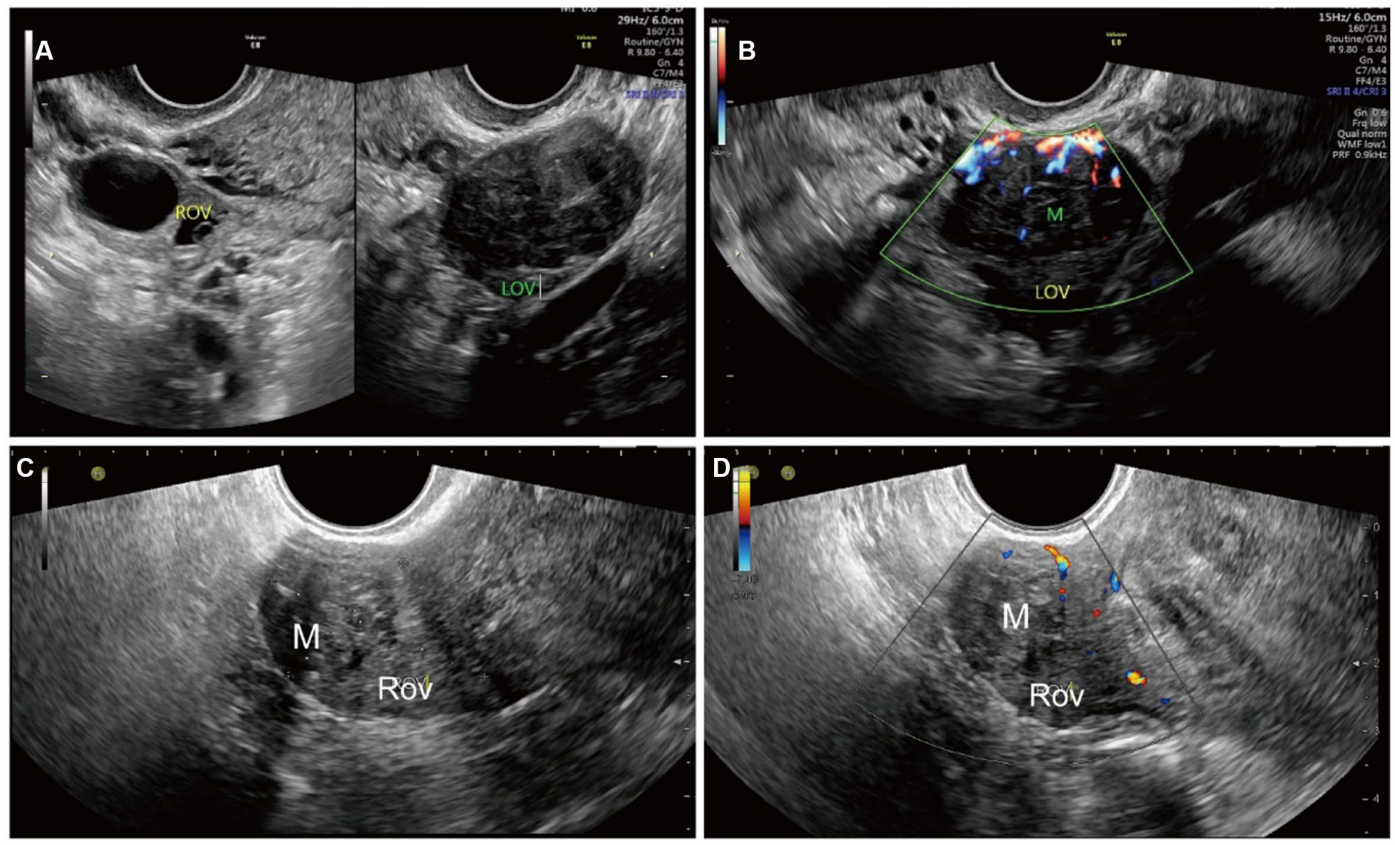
Ultrasonographic imaging of an OL localized inside the ovary in case 4 (A,B) and case 2 (C,D). (A) A well-circumscribed, heterogeneous ovarian mass was detected by transvaginal ultrasound. The mass was hypoechogenic and more hypoechoic than the surrounding ovary. (B) Strip-color flow signals in and around the mass were detected by color Doppler ultrasound. (C) A well-circumscribed, heterogeneous hypoechogenic ovarian mass with echogenicity equal to that of the surroundings was detected by transvaginal ultrasound. The mass was measured to be 19 mm × 17 mm. (D) Strip-color flow signals in and around the mass were detected by color Doppler ultrasound. The patient was treated with laparoscopic mass excision. The patient is alive without postoperative complications or tumor recurrence 1 year after surgery. ROV, right ovary; M, mass.
